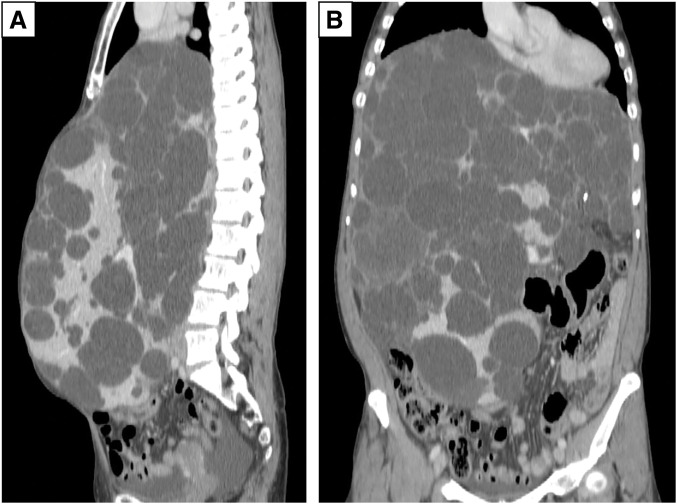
Sagittal and coronal CT images of enlarged cystic liver. (A) Preoperative sagittal CT image. (B) Coronal CT image. The abdominal cavity was filled with a cystic liver, which measured approximately 11.1 L using a 3D image analysis application.
